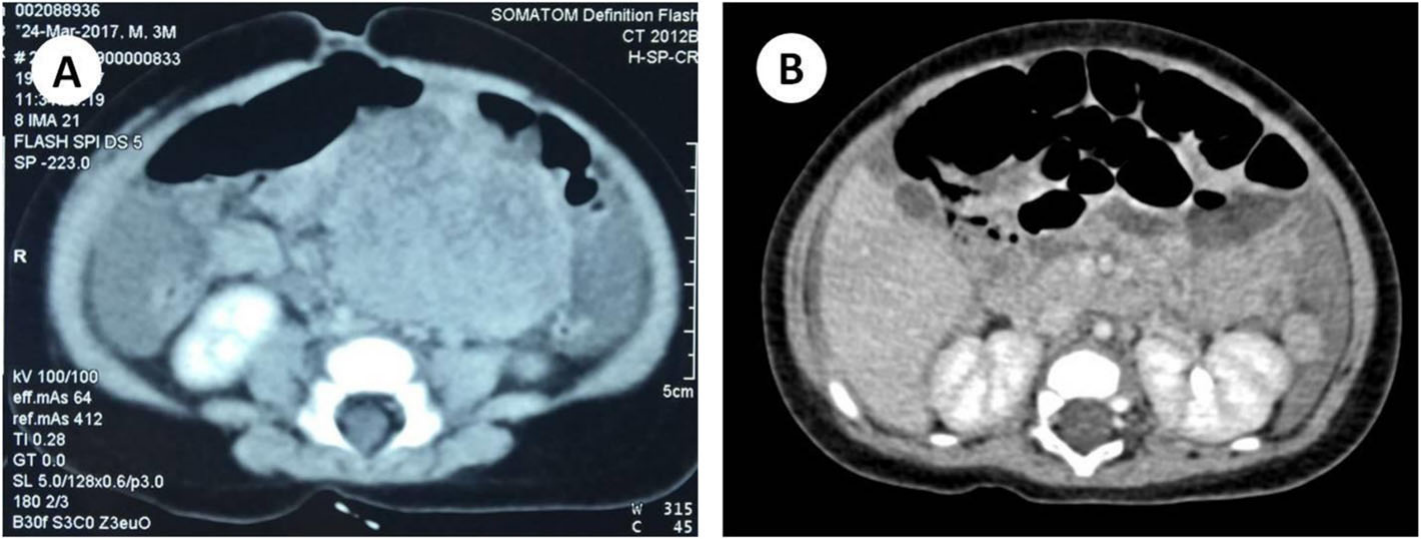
Radiologic findings of the horizontal views. a: Enhanced CT showing a 6.3 × 4.6 cm mass in the left lower retroperitoneal with a clear boarder. b: Enhanced CT findings suggested that multiple metastases were seen in retroperitoneal
